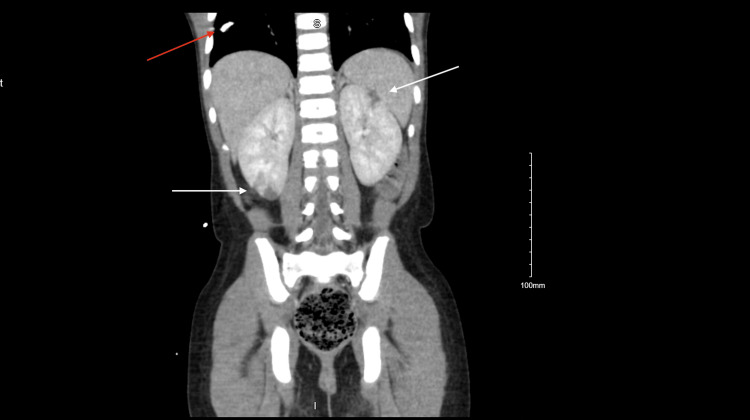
Contrast enhanced CT of the abdomen and pelvis showing right lung base granuloma (red arrow), and bilateral pyelonephritis with multifocal perfusion defects (white arrows)
